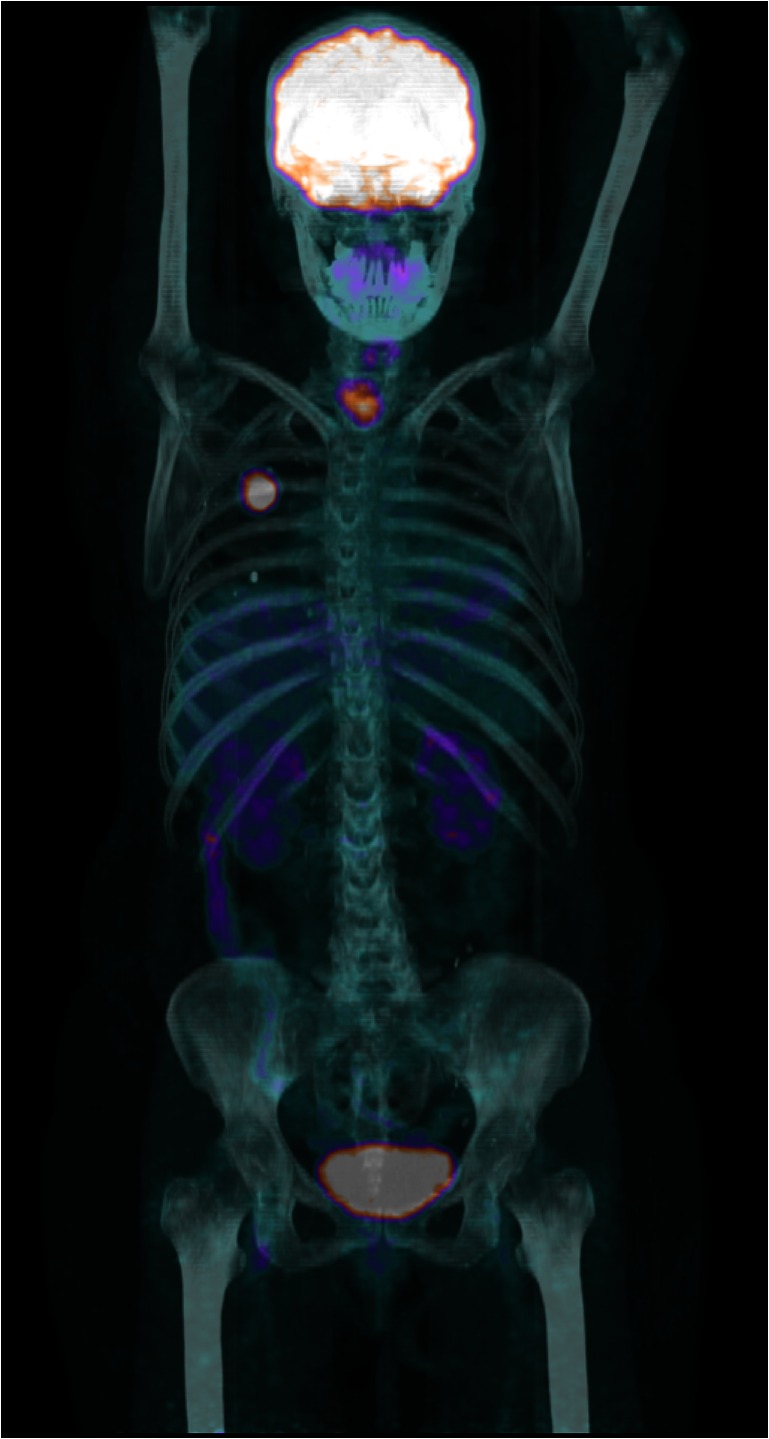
A scan showing one of the powers of PET/CT: In a patient with an isolated tumour in the upper right lobe of the lung, PET/CT revealed a potential lymph node metastasis just above the centre of the right diaphragmatic dome and an unknown lesion in the thyroid gland, not detected during work-up or by other imaging, but verified by biopsy following PET/CT
